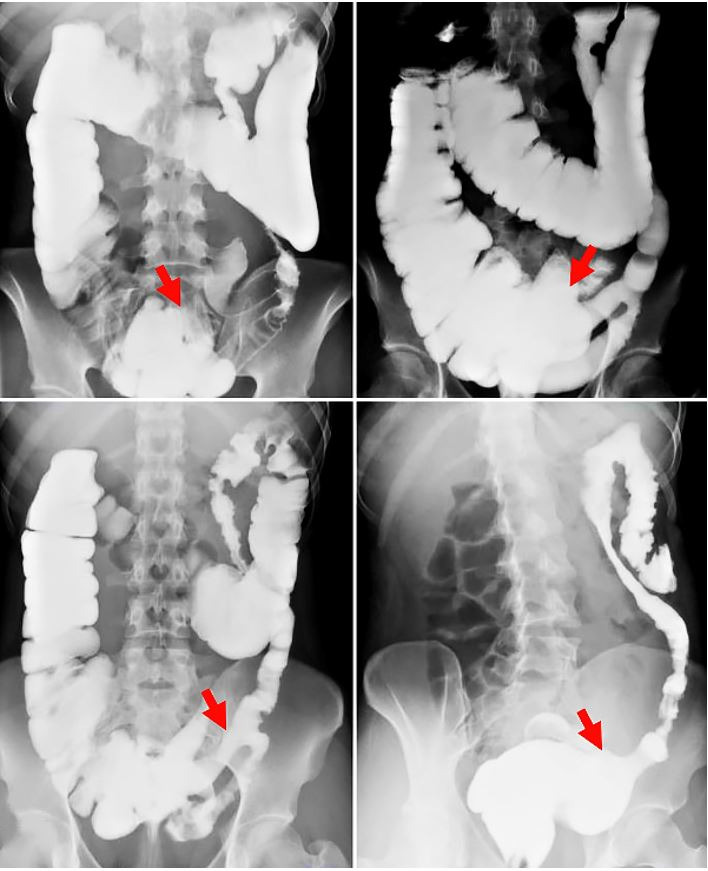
X-ray gastrografin enema. Results showed ahaustral mucosal appearance of the sigmoid and descending colon (red arrows).
